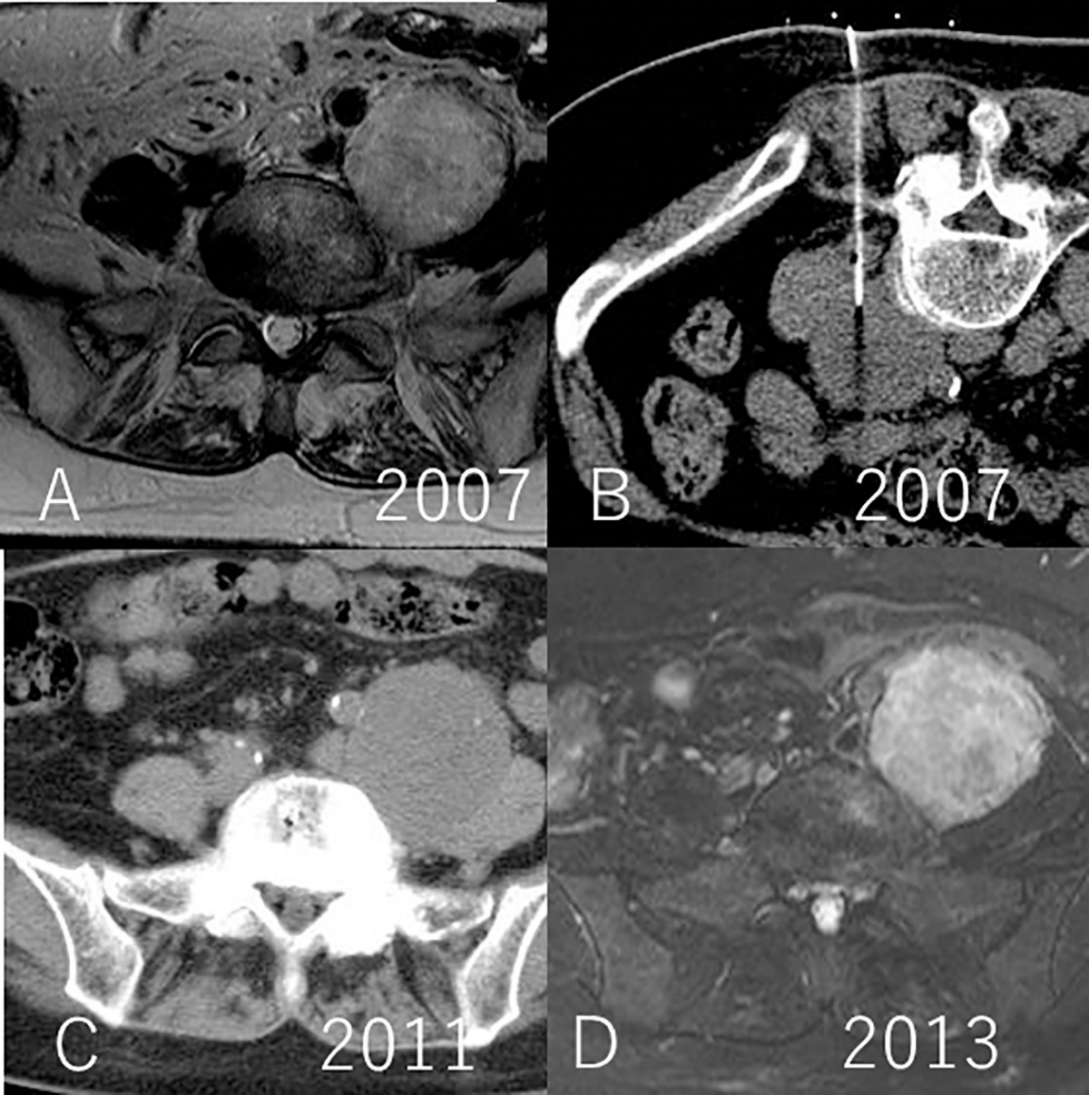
CT and MRI. A. Initial T2 weighted MR image in 2007; B. CT guided needle biopsy in 2007; C. CT scan in 2011; D. T2 weighted MR image with fat suppression in 2013 (case no. 5). Base line diameter of the tumor was 46 mm, and final diameter of the tumor was 58 mm.
